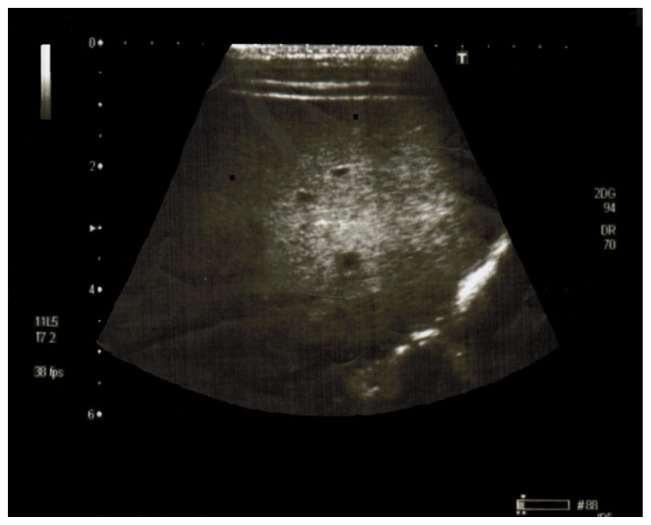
Hepatic ultrasound image 30 days after initiation of corticosteroid therapy.
